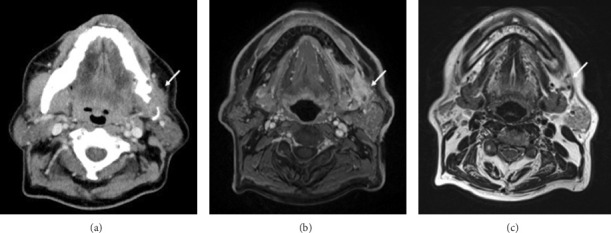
(a) The computed tomography (CT) images. (b, c) The magnetic resonance imaging (MRI) images. The lesions were highlighted by white arrows. CT scan showed a tumor involving destruction of the mandible (a). T1 weighted MRI demonstrated that the tumor with a high signal and irregular margins was observed (b). On T2 weighted MRI, the tumor was low signal, and there was also some signal change in the bone marrow (c).
